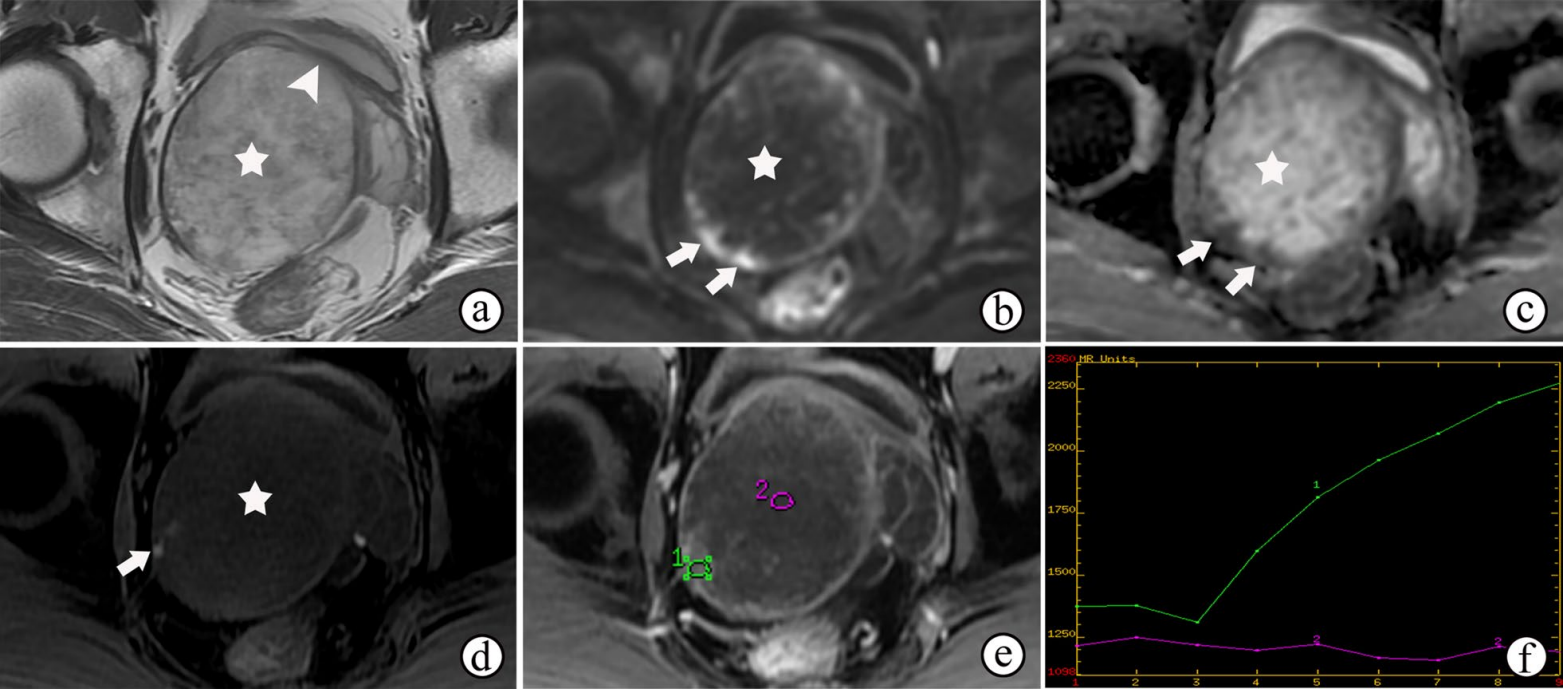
Prostatic stromal sarcoma in a 41-year-old man with a normal serum PSA level of 0.784 ng/mL. a Axial T2WI shows an oval mass occupying the prostate with heterogeneous hyperintensity, an incomplete hypointense capsule and compressing the bladder (arrowhead). b, c Axial DWI and ADC maps show cystic areas occupying the majority of the mass (☆), but containing focal solid components with remarkably restricted diffusion (arrow). d Axial fat-suppressed T1WI shows the mass containing a slightly high signal intensity, which suggested a hemorrhagic change (arrow). (e–f) Axial DCE imaging (e) shows that the mass had diffuse but no enhancement of the cystic components (f, curve 2) accompanied by a gradual moderate enhancement restricted to the solid components (f, curve 1)
